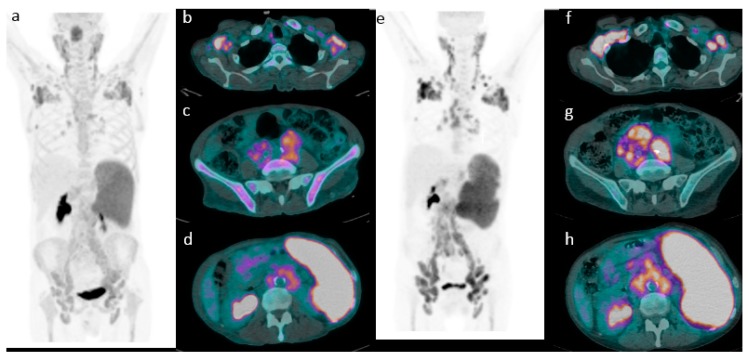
Emblematic example of progressive disease. A representative case of a 65-year-old male with stage III MCL. (a) Baseline maximum intensity projection (MIP, showing diffuse hypermetabolic disease in (b) laterocervical, axillary, (c) iliac and (d) inguinal nodes and in spleen. (e) PET/CT after chemotherapy showing a metabolic progression of disease (Deauville score 5) with the appearance of new lesions (f–h).
